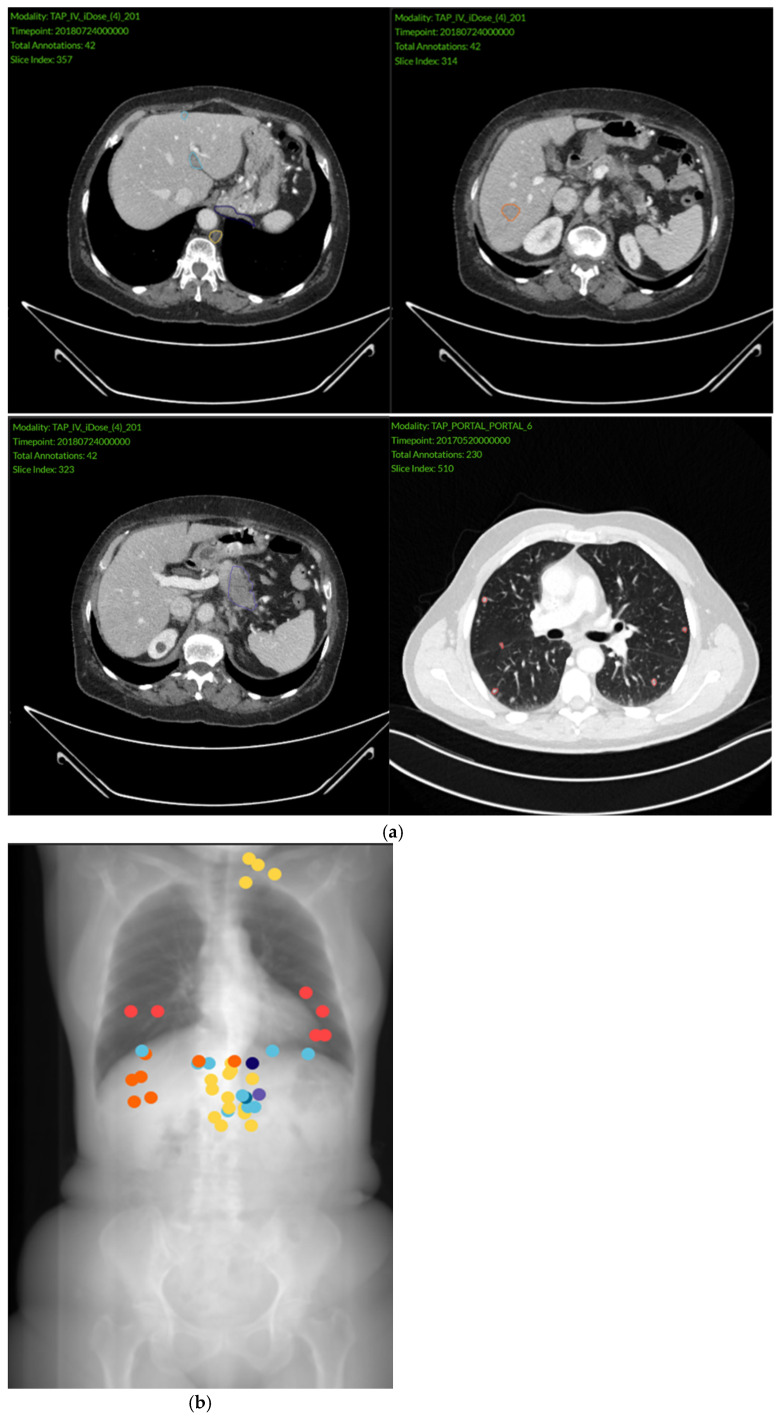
Examples of lesion annotations. (a) An example of annotations of primary and metastatic lesions on CT scan axial slices. Violet = pancreatic tumor, yellow = lymph node metastasis, blue = peritoneal carcinomatosis, light orange = liver metastasis, dark orange = lung metastasis; (b) an example of a scout view of a chest-abdomen-pelvis CT scan with all the annotations labeled by colors according to the tumor location. Abbreviations: CT = computed tomography.
